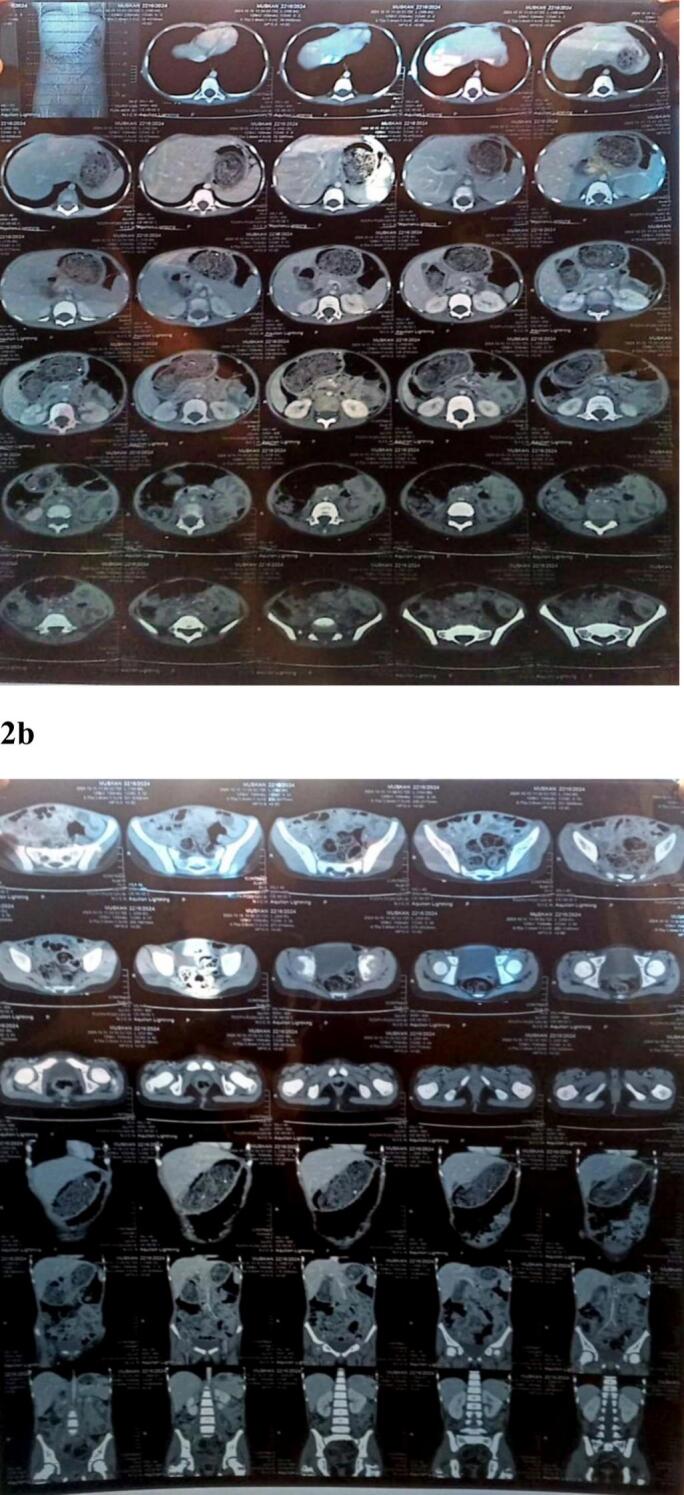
a and b. This is a contrast-enhanced CT scan (axial and coronal views) that revealed a large gastric trichobezoar with extension into the duodenum and proximal jejunum, consistent with Rapunzel syndrome. Mild proximal small bowel dilatation suggested jejunojejunal intussusception with a lead point of mesenteric lymphadenopathy, with no signs of perforation or ischemia.
